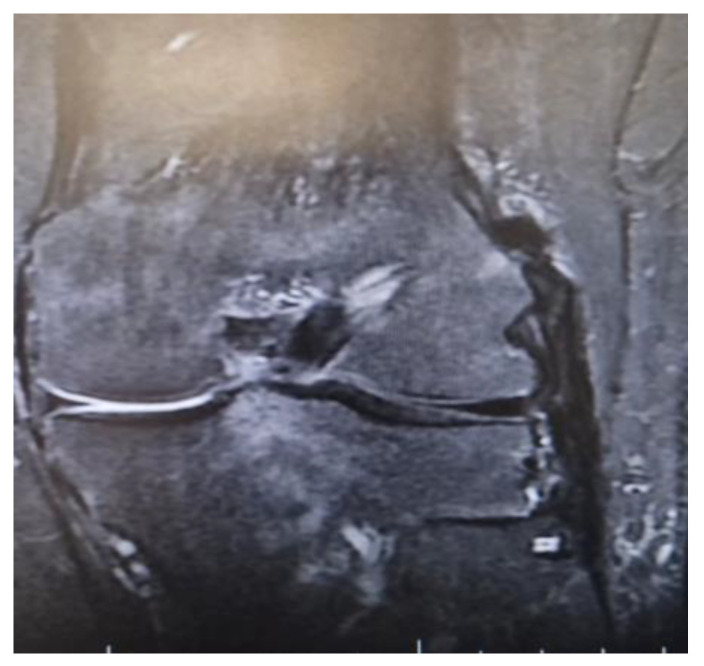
MRI demonstrating a single Achilles allograft used to reconstruct ACL, LCL, and ALL, with clear visualization of the graft distribution.
